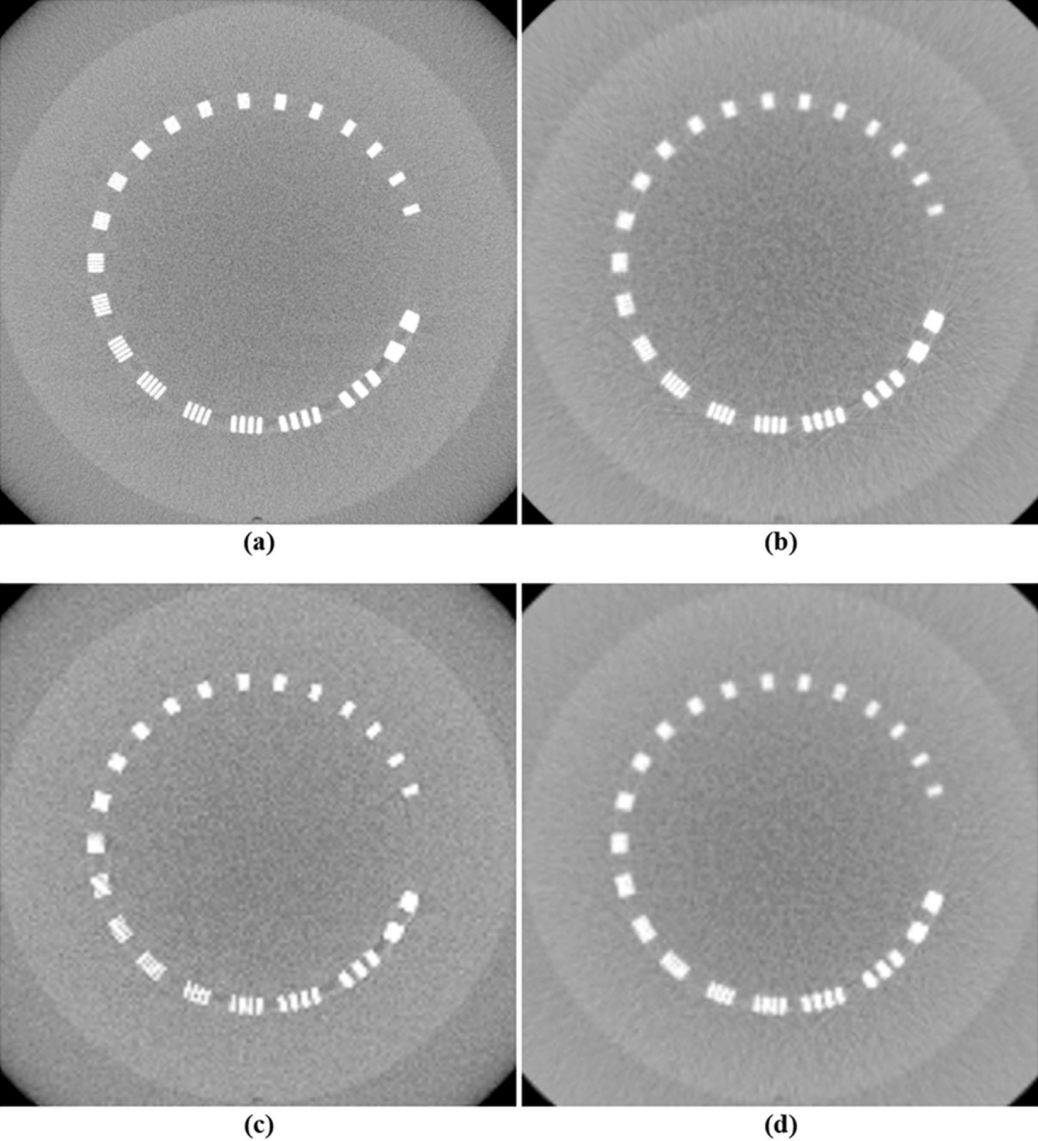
Comparison of a representative slice of the reconstruction image generated by applying FDK using high mAs projections and FDK with NLTV + cubic B-spline interpolation using low mAs projections. (a) high resolution benchmark image, (b) high resolution CBCT produced by the proposed method, (c) low resolution benchmark image, and (d) low resolution CBCT produced by the proposed method. These images are displayed at window (width and level) settings of (2000, 0) HU.
